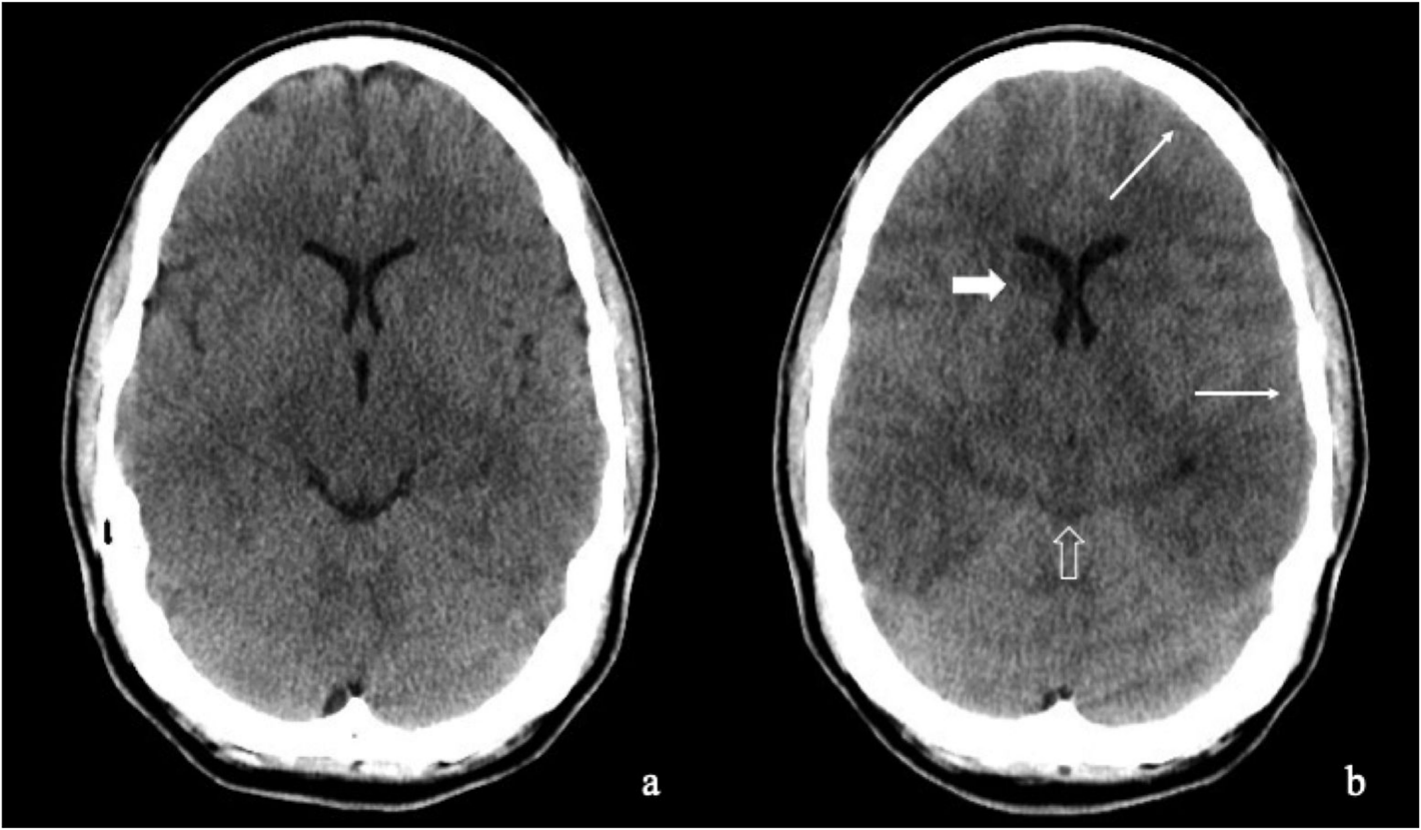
An axial non enhanced computed tomography (NECT) scan performed on the first day (a) and 24 h later (b) show the appearance of diffuse cerebral edema with initial loss of normal gray-white matter differentiation (narrow white arrows), obliteration of CSF spaces (unfilled think arrow) and the “disappearing” of the basal ganglia (i.e., head of the right caudate nucleus) (filled thick arrow)
